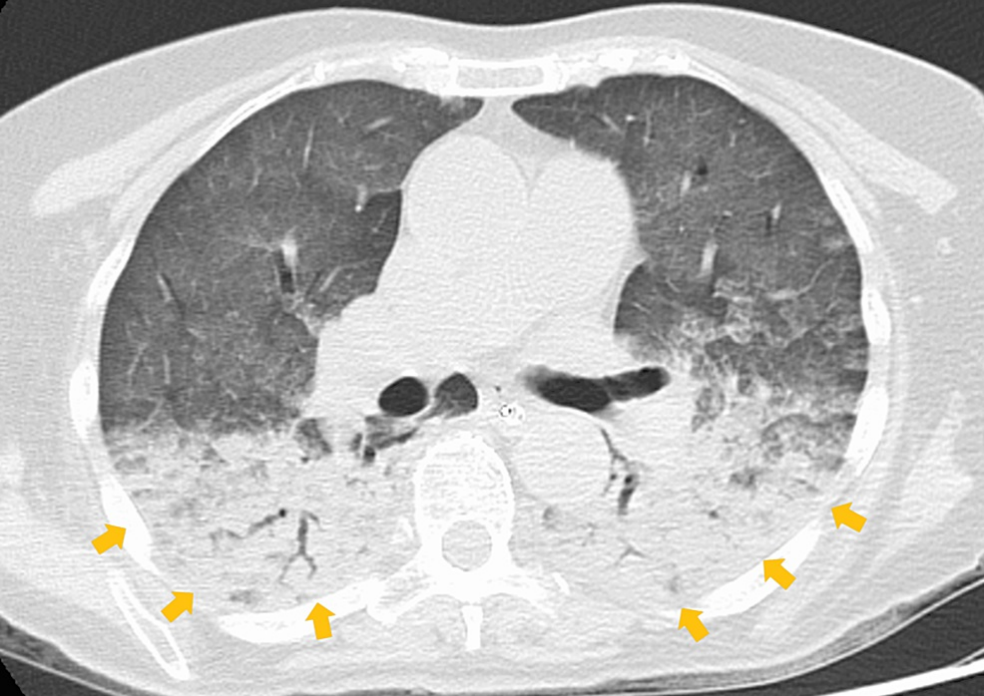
Computed tomography showing bilateral lung infiltrates Chest HRCT shows bilateral infiltrating shadows with air bronchograms in the dorsal lungs (arrows). Both lungs also showed diffuse ground-glass attenuation due to gravity. No cardiac enlargement was observed. These findings are consistent with those of ARDS due to drowning. HRCT: high-resolution computed tomography, ARDS: acute respiratory distress syndrome
